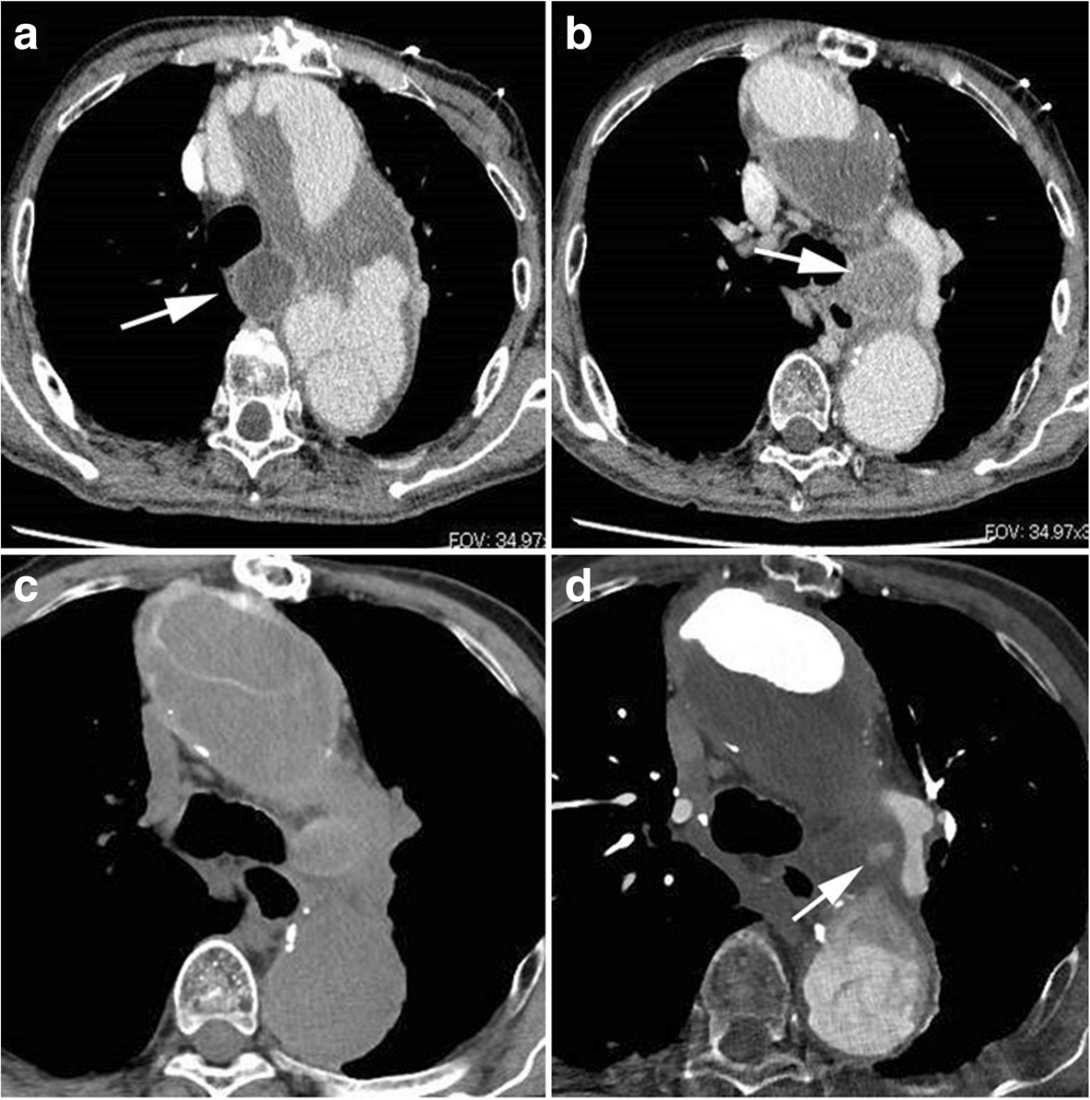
A 76-year-old male with massive hemoptysis. a and b. CT showed two aortic pseudoaneurysms (arrows), which were in contact with the aortic anastomoses. They were attached to the trachea and left bronchus, respectively, which was suggestive of AB fistula formation. c and d. Two months before a and b, CT showed that an aortic pseudoaneurysm had blood flow (arrow). After that, the psuedoaneurysn increased in size
